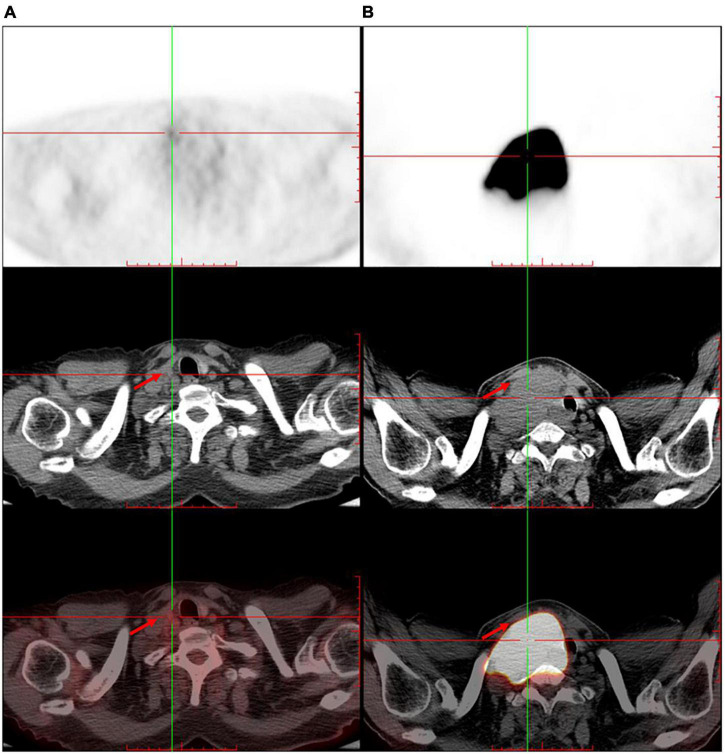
The trunk positron emission tomography/computed tomography shows significant shrinkage of the original large mass in the right neck after four cycles of immunochemotherapy. (A) After immunochemotherapy for four cycles. (B) Before the immunochemotherapy. Red arrow indicates the tumor.
